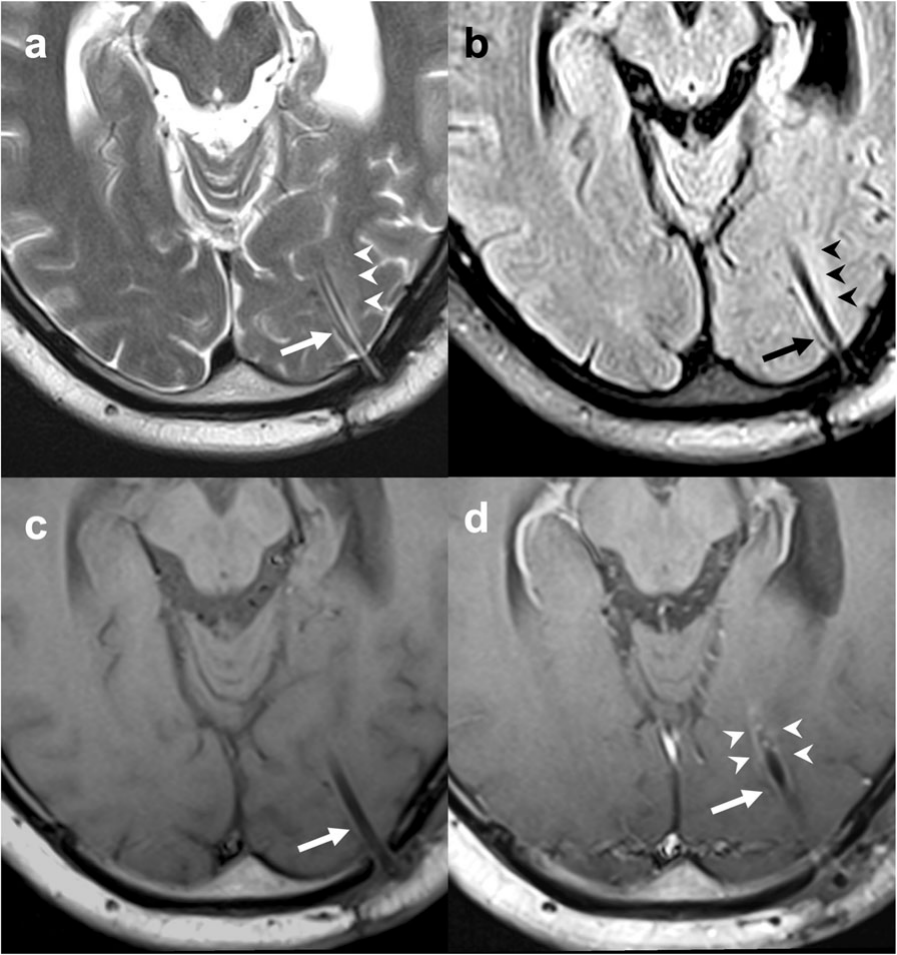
Ventricular catheter. Axial T2-weighted (a), FLAIR (b), unenhanced (c), and post-contrast (d) T1-weighted images presenting a left occipital access ventricular catheter (arrows) with mild peripheral gliosis (arrowheads in a and b) and subtle enhancement (arrowheads in d)
